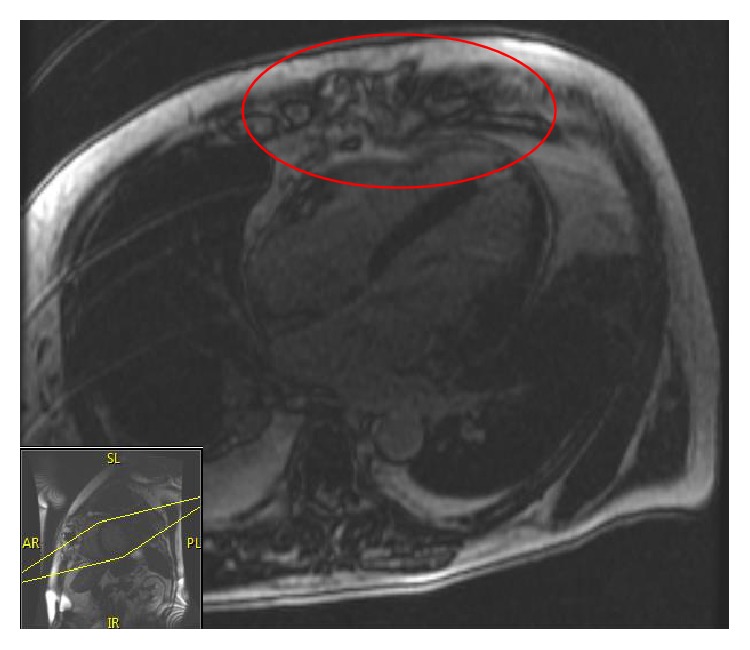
Cardiac MRI (four-chamber view) demonstrates a 1.5 cm small abscess contiguous with pericardial enhancement (red oval). Inset at bottom left demonstrates plane of cross-sectional image (SL = superolateral, PL = posterolateral, AR = anterior right, and IR = inferior right).
